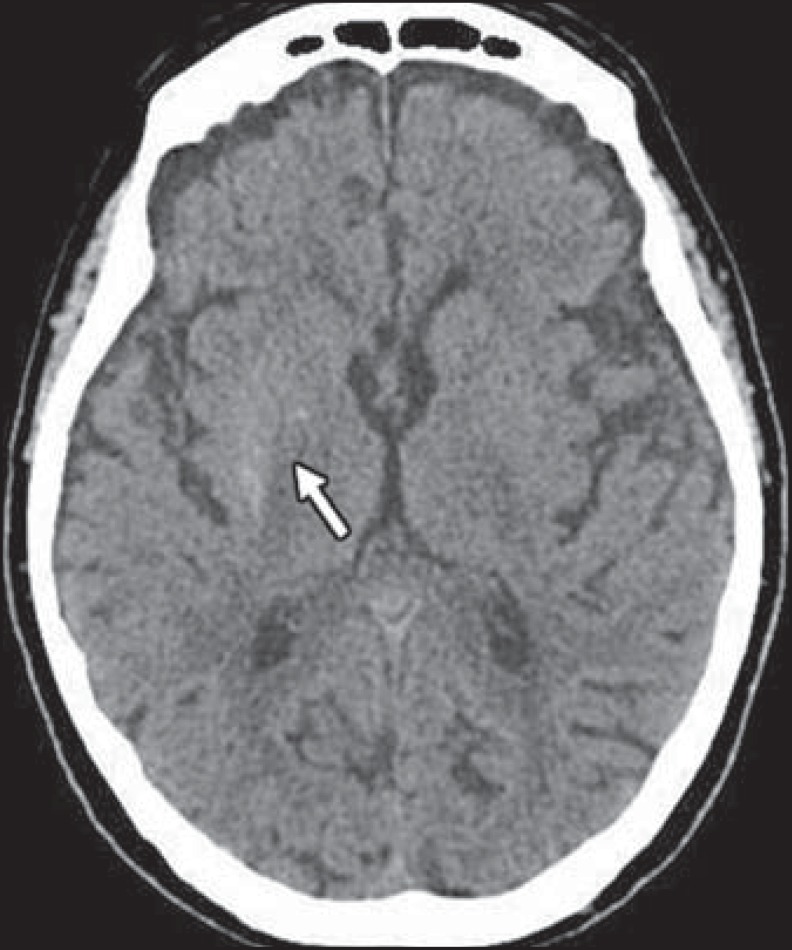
Axial unenhanced brain CT scan, acquired at hospital admission, showing
